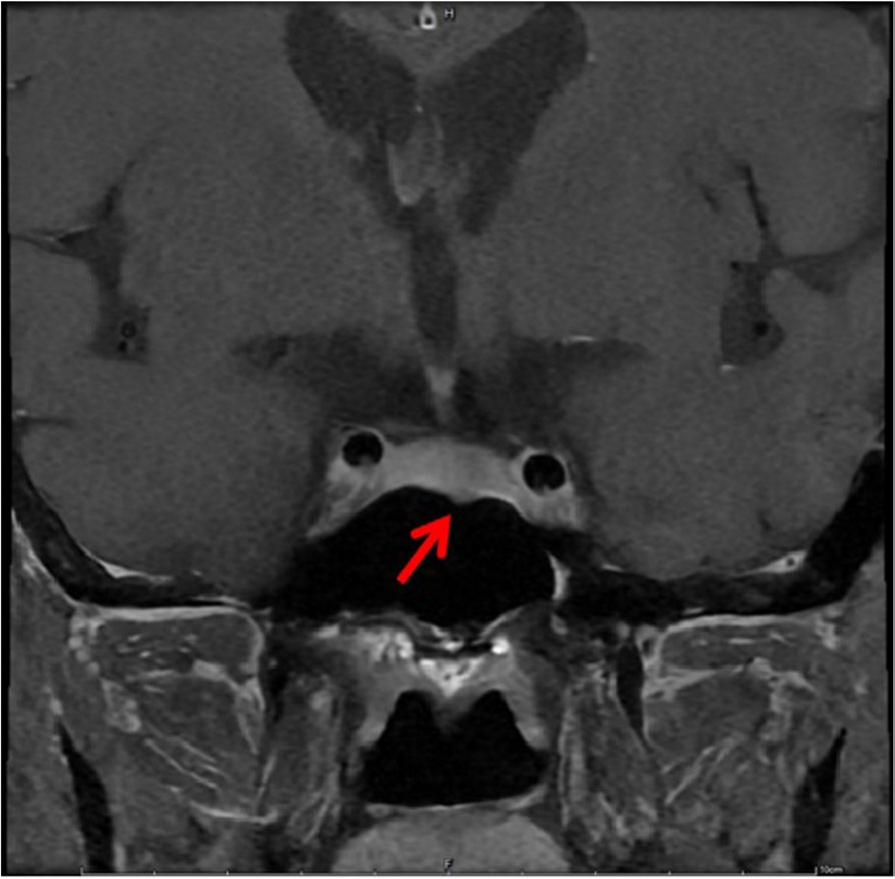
A pituitary microadenoma (0.4 cm) was identified using magnetic resonance imaging (arrowhead)
